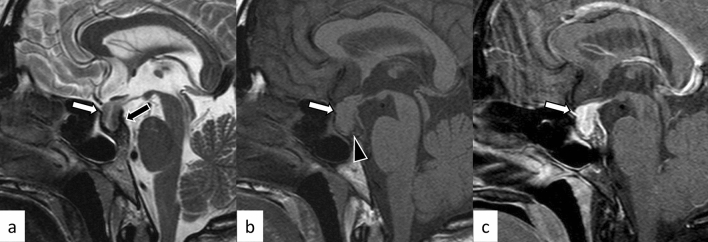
A 38-year-old woman with lymphocytic hypophysitis. An enlarged pituitary gland shows heterogeneous hyperintensity on T2-weighted sagittal image (a, white arrow), isointensity on T1-weighted sagittal image (b, white arrow), and homogeneous contrast enhancement (c, white arrow). A dark signal intensity area is observed on the sellar floor (“parasellar T2 dark sign”; a, black arrow). The posterior pituitary T1-weighted bright spot is absent (b, arrowhead). The different images of this case were evaluated in the previous study [9]
